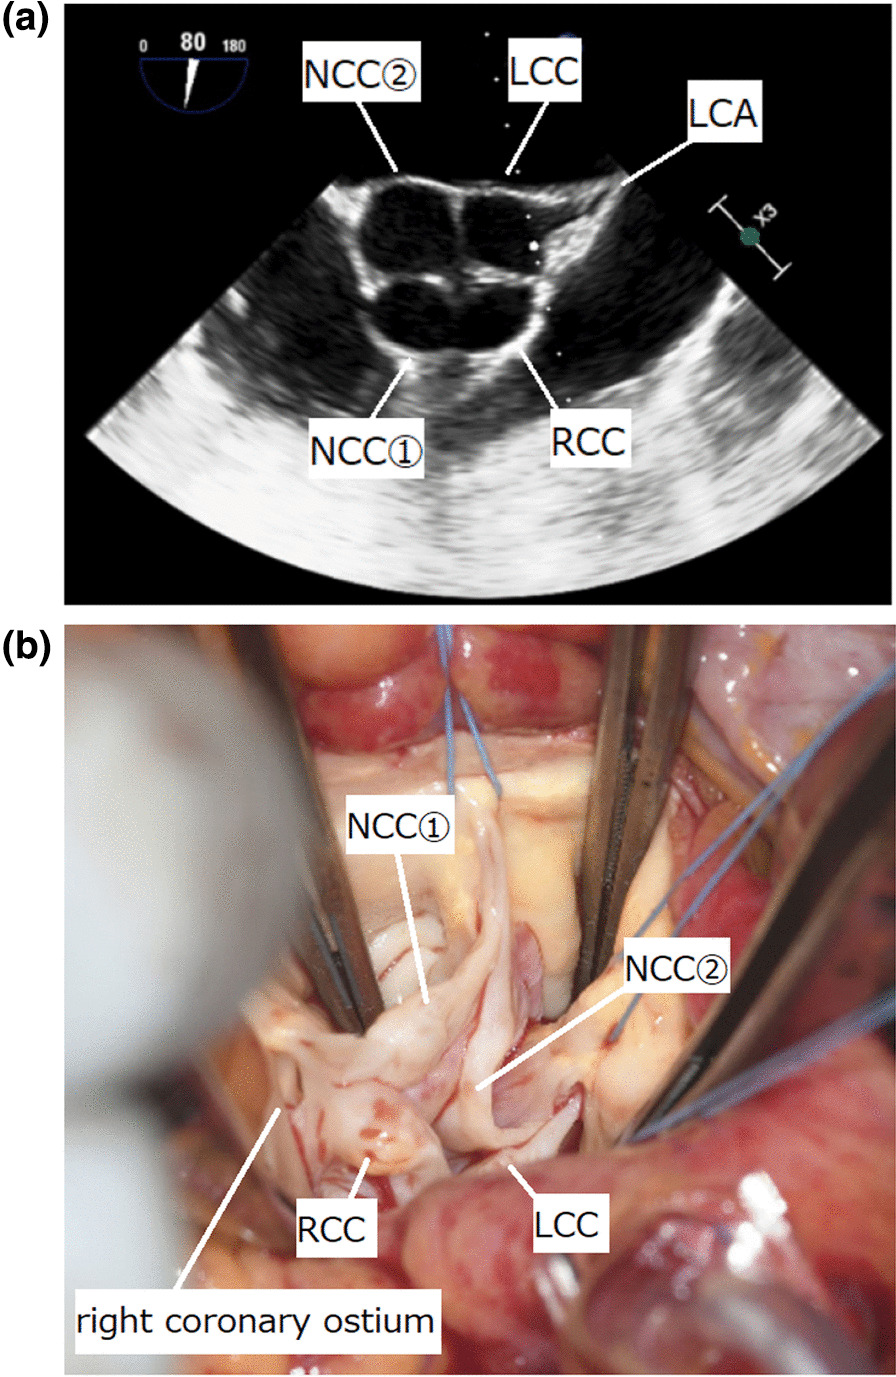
a Intraoperative transesophageal echocardiography showing almost equal-sized QAV (Hurwitz and Roberts classification type A). b Intraoperative photograph showing the QAV. The right coronary ostium is located adjacent to the commissure between the right coronary cusp and one of the two non-coronary cusps. LCA, left coronary artery; LCC, left coronary cusp; NCC, non-coronary cusp; QAV, quadricuspid aortic valve; RCC, right coronary cusp
